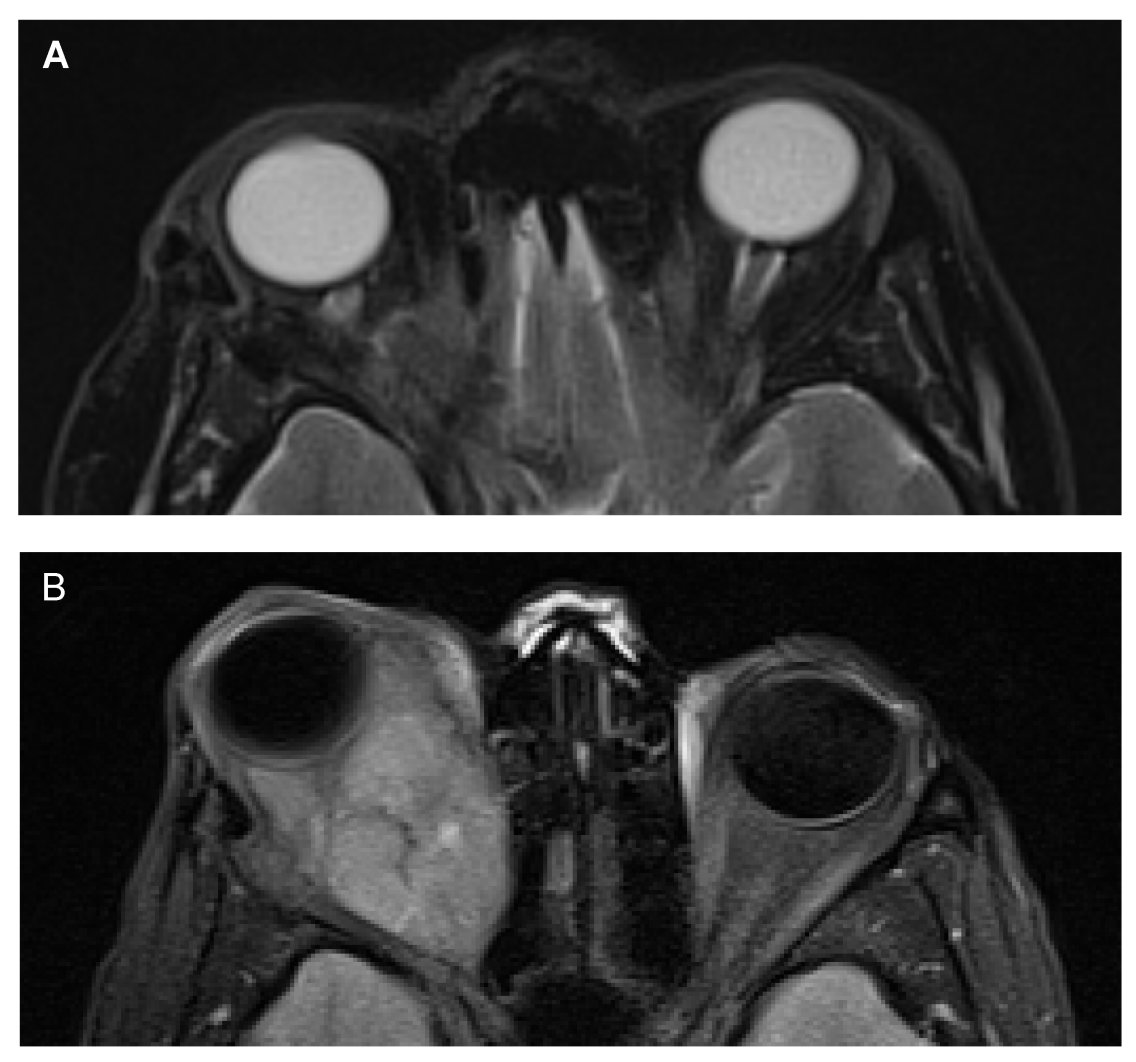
Orbital MRI A) The first post-surgical MRI showed a blue nevus remnant in the right orbit. B) Follow-up MRI approximately 5 years after initial presentation showed a lobulated posterior right orbital mass causing a significant proptosis that was pathologically described as a blue nevus with areas of high and low-grade melanoma.
